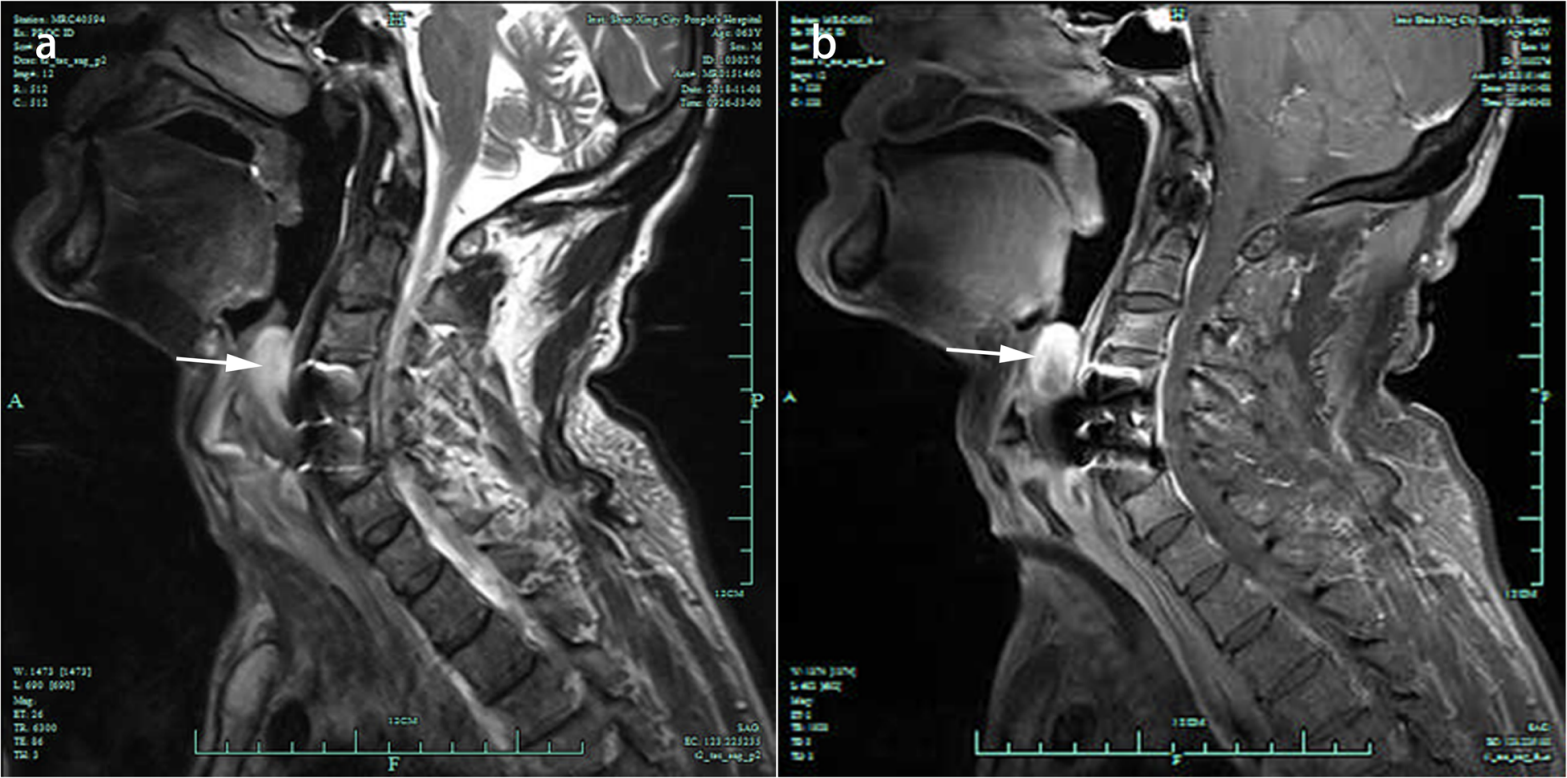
Images of Cervical MRI. a, b T2-weighted sagittal images revealed an oval mass of high-signal mass (white arrow) that completely blocked the throat
